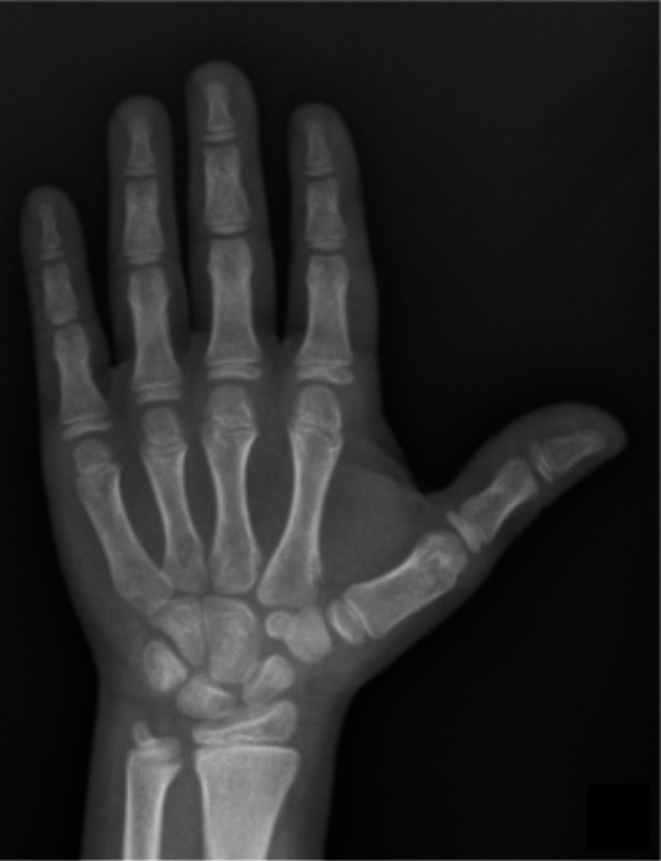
Radiograph of the left hand and wrist of a 10‐year‐old female patient with hypochondroplasia, showing reduced phalangeal and metacarpal lengths of less than 2 SD; however, this alteration is not readily apparent on a cursory analysis.
